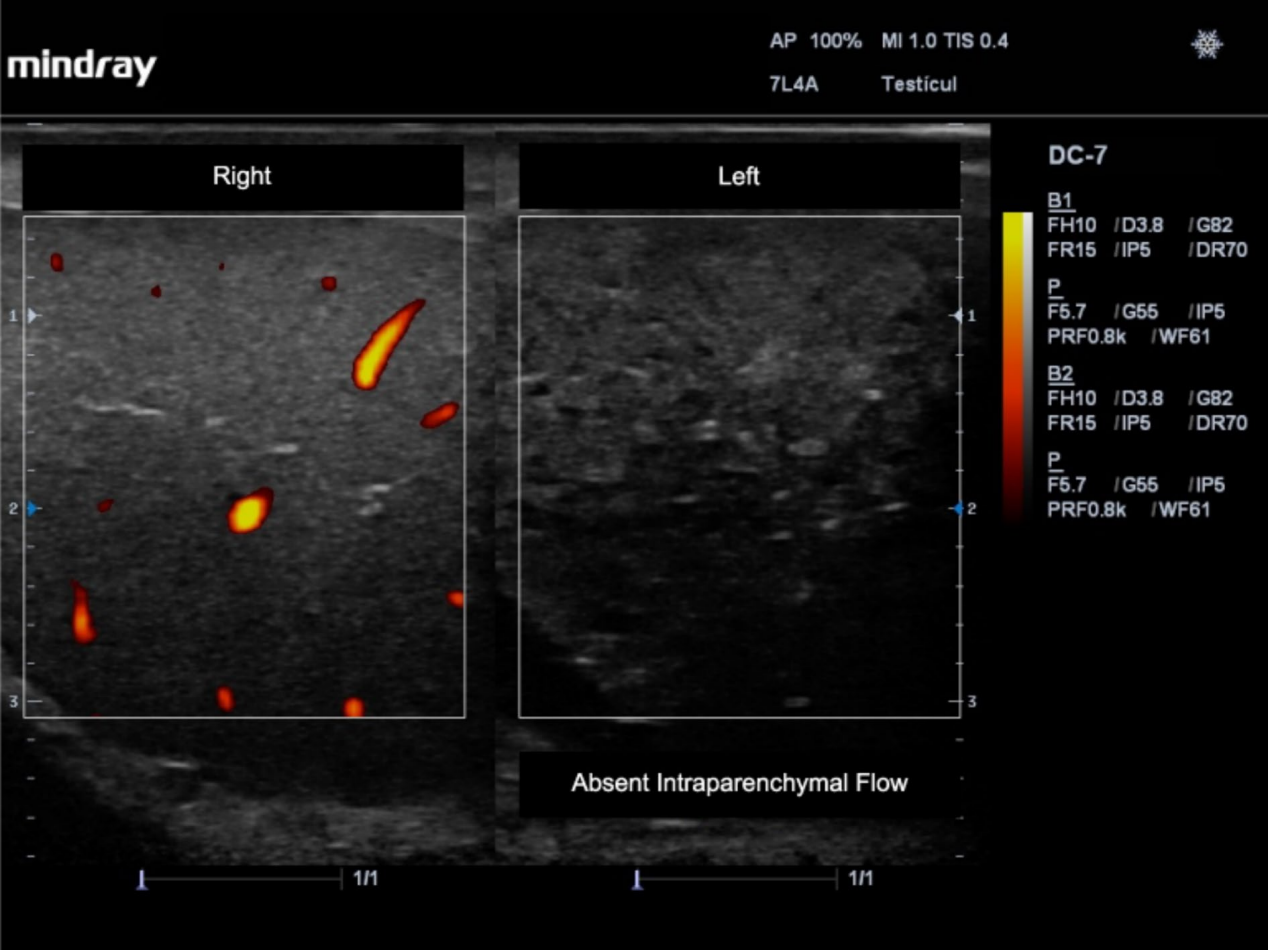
Absent flow and changes in echogenicity in the left testicle due to testicular torsion. Power Doppler was applied and a comparison with the right non-affected testicle is shown
